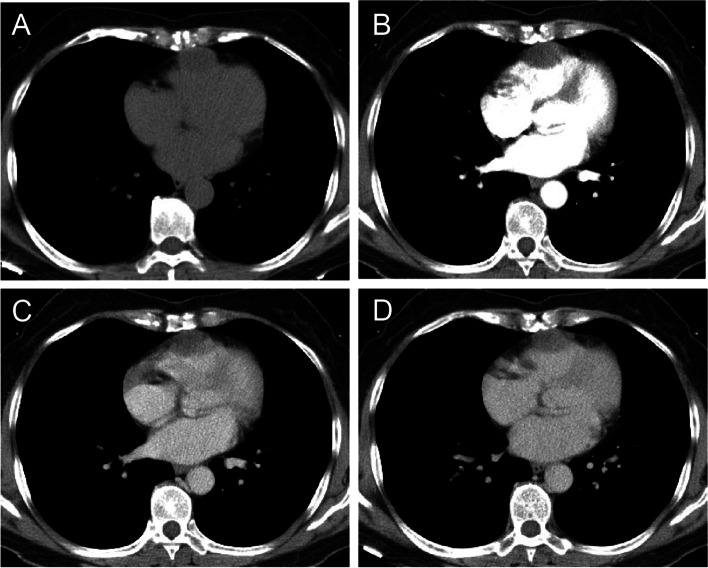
A An isodense mass on the anterior wall of the right ventricle with uniform internal density and clear boundary. No obvious narrowing of the heart cavity was notede, the CT value is approximately 29 HU. B A myocardial tissue mass with slightly enhancement during the arterial phase, the CT value is approximately 34 HU. C-D Lesions with a persistent enhancement during the venous and delayed phase, the CT value is approximately 38 HU (C) and 35HU (D)
